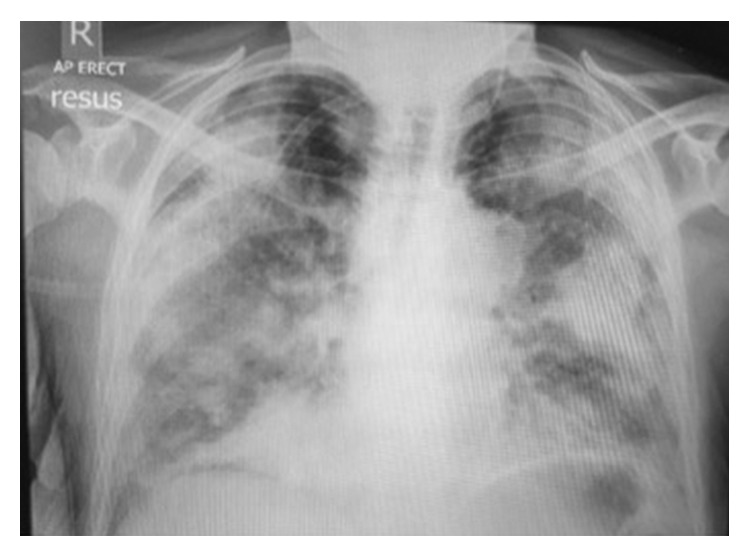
Chest radiograph showing multiple foci of consolidation representing multifocal pneumonia or possible pulmonary infarcts secondary to multiple pulmonary emboli.
